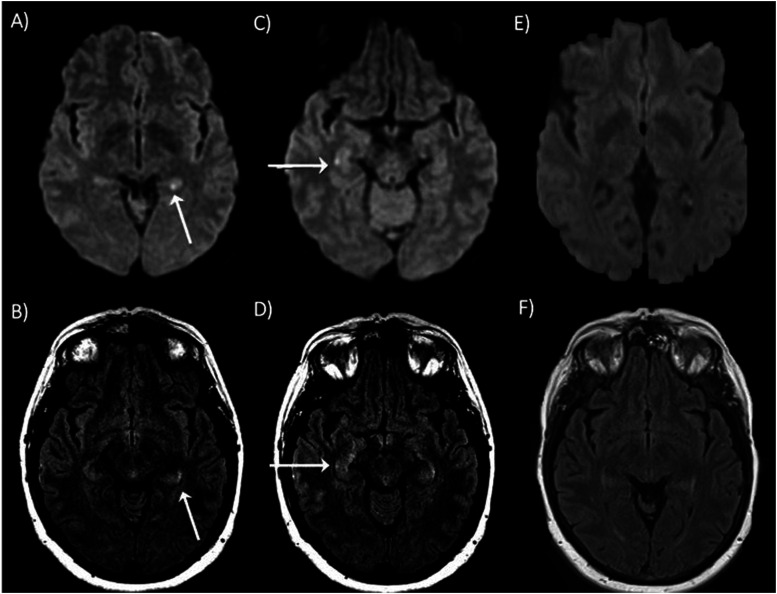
Diffusion weighted imaging (DWI) changes in a patient with suspected transient global amnesia. On initial brain magnetic resonance imaging (MRI) 2 punctate foci of restricted diffusion were seen in the hippocampi bilaterally. The left-sided DWI lesion was in the lateral aspect of the hippocampal head (A), with corresponding hypointensity on apparent diffusion coefficient (ADC, not shown in this figure), and hyperintensity on fluid attenuated inversion recovery (FLAIR, B). A right-sided DWI lesion was positioned more posteriorly towards the hippocampal body (C), with corresponding hypointensity on ADC, and hyperintensity on FLAIR (D). A repeat MRI 6-months later showed complete resolution of the punctate foci of diffusion restriction (E, F).
